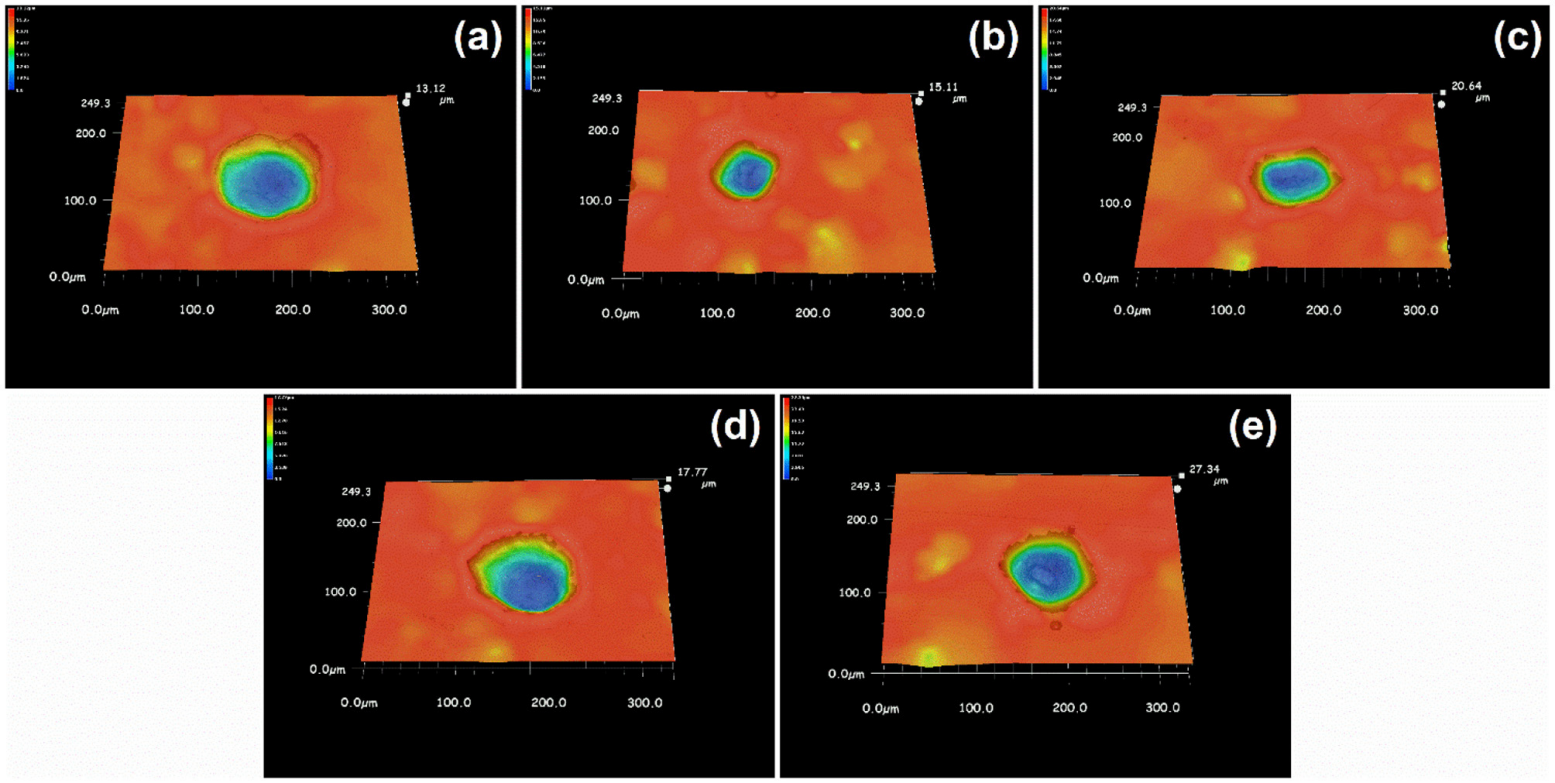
The 3D profile of single pits after ultrasound treatment after polarization tests terminated at 100 μA cm^−2^ in 1 M NaCl solutions with (a) 0 M, (b) 1 M, (c) 2 M, (d) 5 M, (e) 10 M ethanol.
